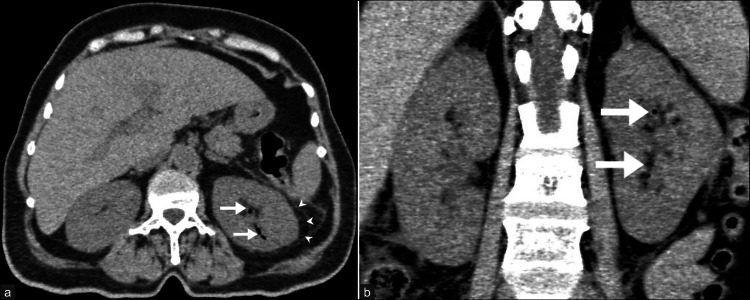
Early emphysematous pyelonephritis. A 62-year-old woman with a history of fever, vomiting, and abdominal pain was diagnosed with early emphysematous pyelonephritis. (a) Non-contrast axial CT of the abdomen image shows a few air foci (arrows) in the parenchyma and pelvicalyceal system of the left kidney, along with minimal perinephric fat stranding. (b) Coronal reformatted image of the left kidney shows the extent of air foci. These images are from the hospital and have not been published elsewhere. CT: computed tomography
